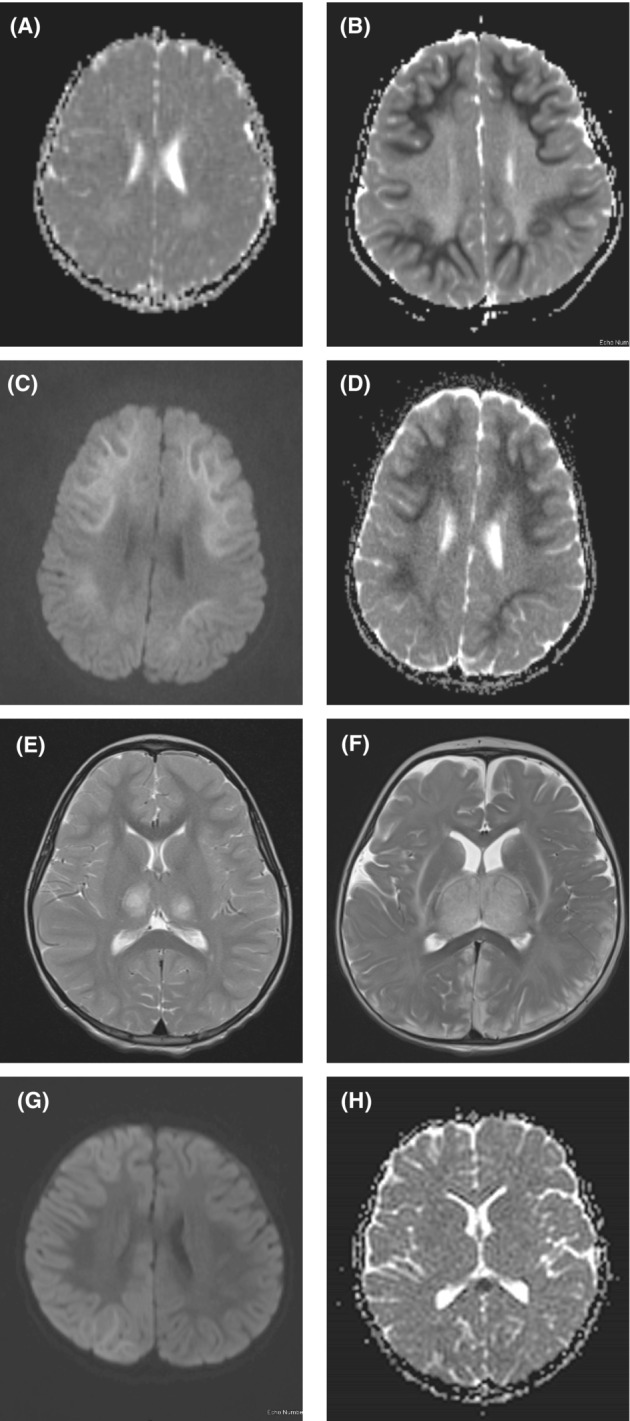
MRI scanning of representative ITES cases. Axial ADC MRI showing normal scan in Case 3 (AESD) on Day 2 (A), and axial ADC MRI showing restricted diffusion of white matter with sparing of peri‐rolandic regions on Day 6 (B). Axial DWI MRI showing increased signal in Case 2 (AESD) on Day 5 (C) and axial ADC showed diffusion restriction (D) in same patient. Axial T2‐weighted MRI in Case 10 (ANE) on Day 2 shows bi‐thalamic lesions (E) which were diffusion restricted on ADC imaging. Axial T2‐weighted MRI in Case 9 (ANE) on Day 2 shows bi‐thalamic lesions which are swollen, plus involvement of the white matter, capsules and cerebral cortex (posterior predominant) (F). Axial diffusion‐weighted imaging MRI of Case 11 (HHE) on Day 3 shows cortical and subcortical brightness of the right hemisphere (G). Axial ADC MRI of Case 12 (MERS) on Day 2 shows diffusion restriction of the splenium of the corpus callosum (H).
